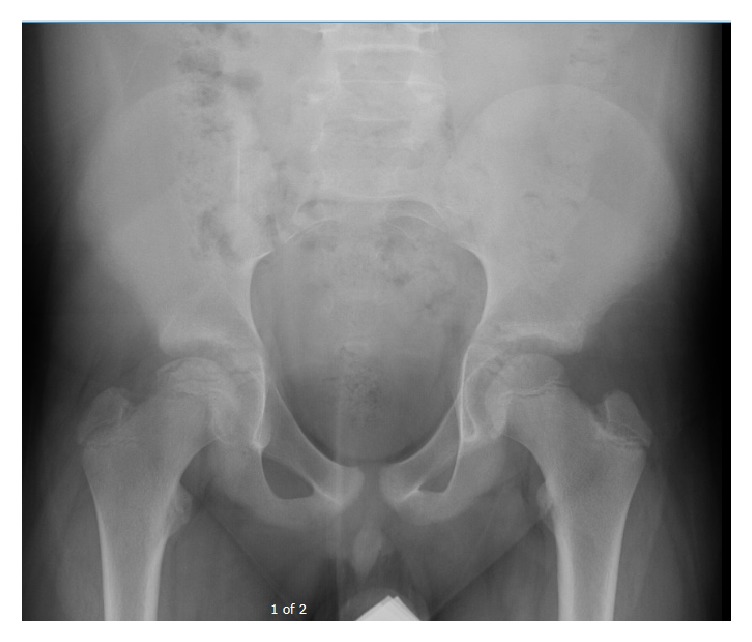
AP pelvis X-ray showing avascular changes of subchondral cystic lucency without evidence of femoral head collapse.
